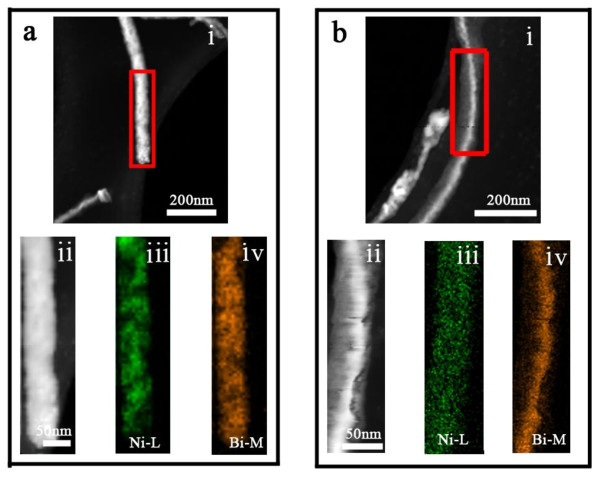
Images of a Bi-Ni nanowire (a) and nanocable (b) obtained using STEM. STEM HAADF images of (i) a Bi-Ni nanowire and nanocable and (ii) after an EDS image scan of the marked area in (i). Elemental mapping of the (iii) nickel and (iv) bismuth X-ray intensities compositing along the nanowire and nanocable.
